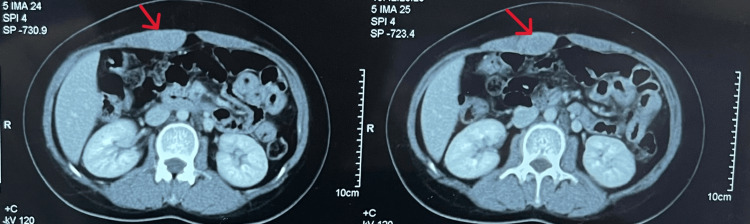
A contrast-enhanced CT scan shows a mass within the right rectus sheath
